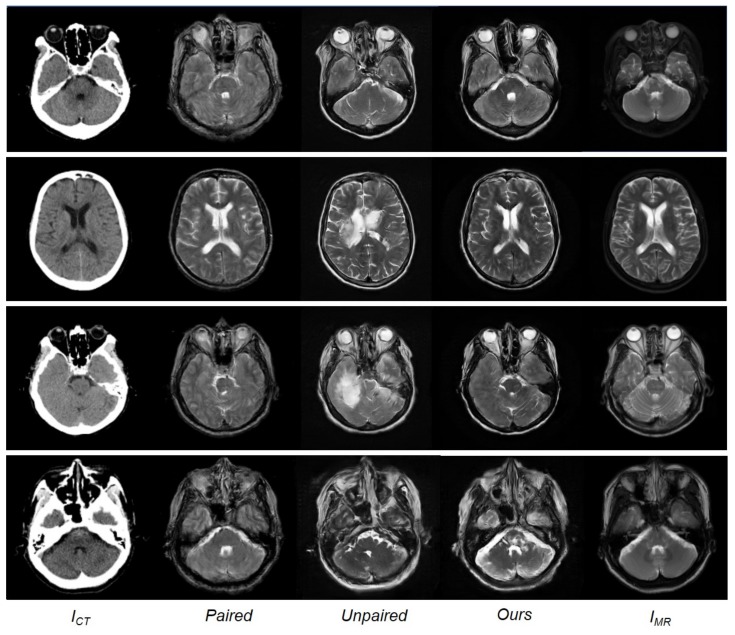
From (left) to (right): Input CT image, synthesized MR image with paired training, synthesized MR image with unpaired training, synthesized MR images with paired and unpaired training (ours), and the reference MR images.
